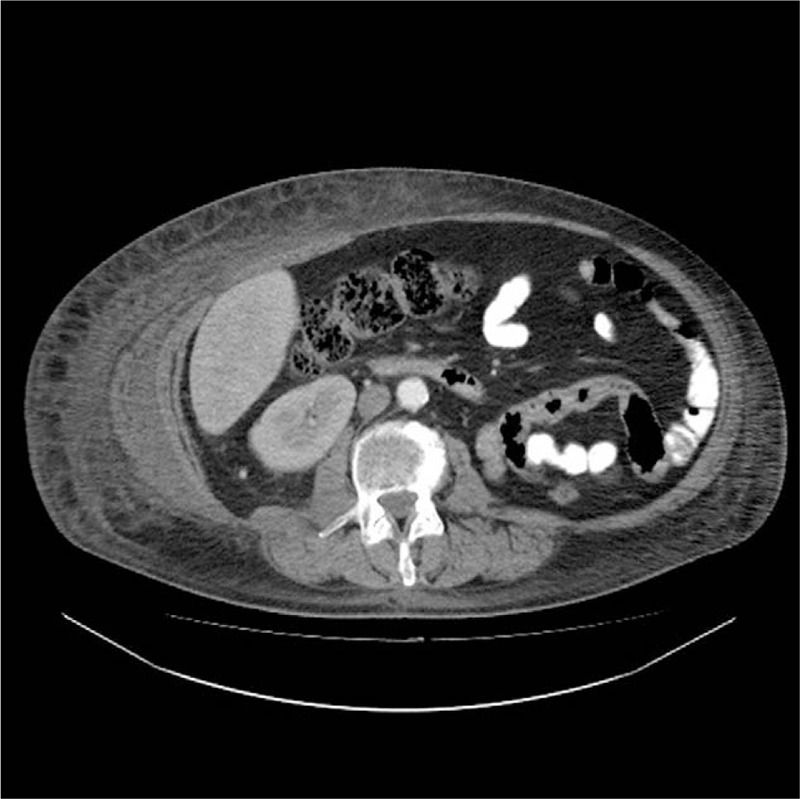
Axial contrast-enhanced computed tomography of lymphedema on the right side of the abdomen.
